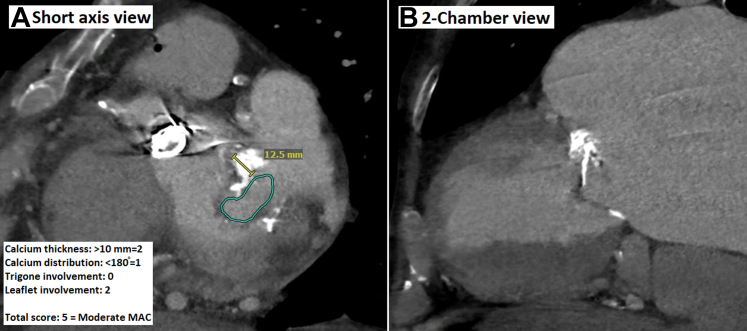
Computed Tomography of the Chest Showing Moderate MAC (A) Short-axis view. (B) Two-chamber view. MAC = mitral annular calcification.
